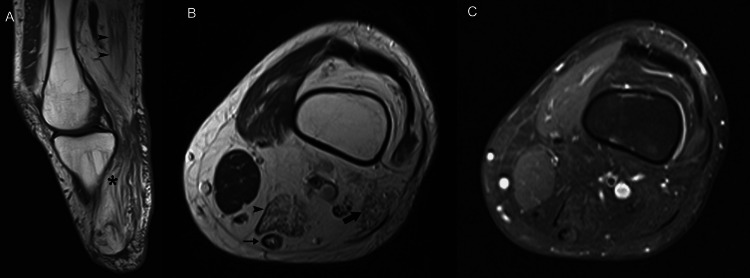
A 48-year-old man after remote below-knee amputation. (A) Sagittal T1-weighted MR image shows increased signal consistent with fatty infiltration of atrophied semimembranosus (arrowheads) and gastrocnemius muscles (asterisk) due to chronic disuse. (B) Axial T2-weighted MR image shows fatty infiltration pattern involving semimembranosus (arrowhead), semitendinosus (arrow), and biceps femoris (thick arrow) muscles. (C) Corresponding T2-weighted MR image with fat suppression reveals a lack of high-signal-intensity fat within infiltrated muscles.
